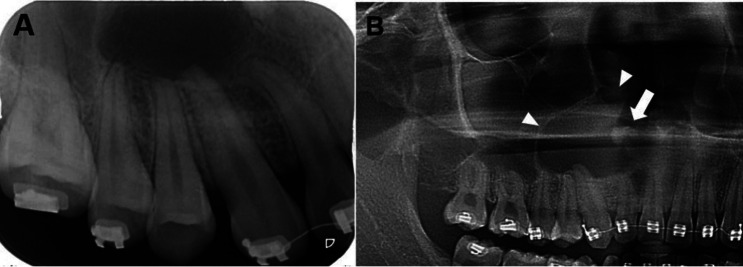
Radiographs of a dentigerous cyst. A. A periapical radiograph reveals a well-defined radiolucent lesion associated with apical regions of upper right lateral incisor to the second premolar, leading to the misdiagnosis of endodontic lesion. B. A cropped panoramic radiograph of the same patient reveals entire part of the lesion which is associated with an impacted supernumerary tooth (arrow). The floor of the right maxillary sinus and nasal cavity are elevated by the lesion (arrowheads).
